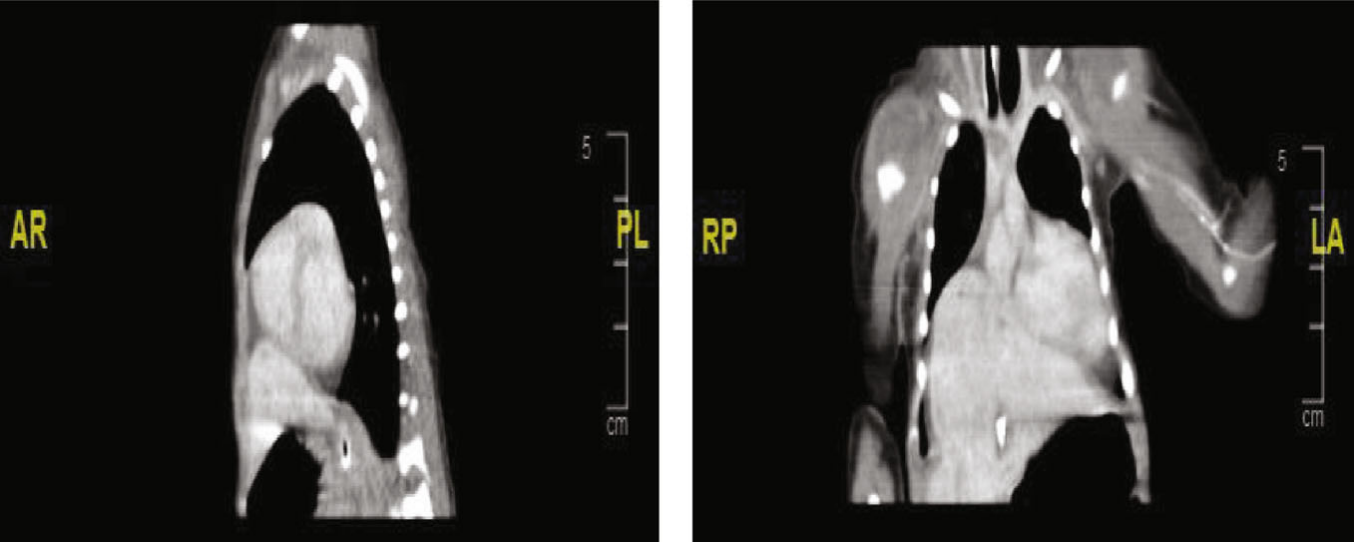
Preoperative computed tomogram cuts, showing the herniated liver into the thoracic cavity, large pericardial effusion, and compressed lungs.
